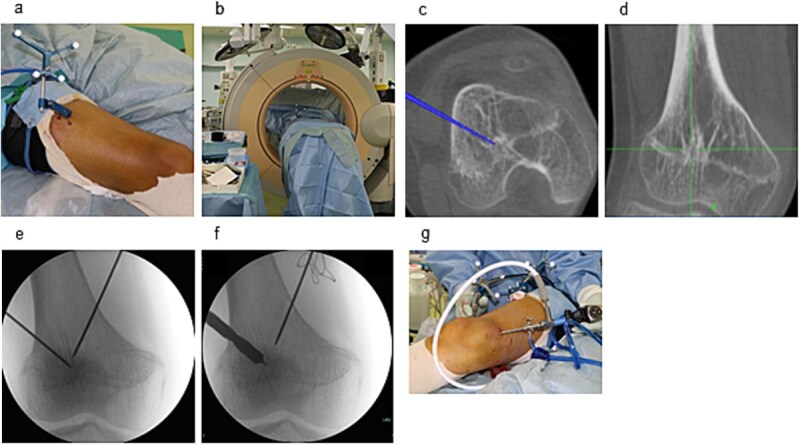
Intraoperative setup and portal creation. (a) Two 3-mm pins were inserted into the femoral diaphysis to secure the tracker of the Stealth Station™ system. (b) Intraoperative CT was performed using the O-arm™ system and integrated with the navigation system. (c) The location and trajectory to the physeal bar were identified using navigation. (d) Two 1.6-mm Kirschner wires were inserted from the medial and lateral aspects toward the physeal bar under fluoroscopic guidance. (e) Drill portals (8 mm) were created along the wire tracts. (f) A 4-mm arthroscope was inserted through one portal for endoscopic visualization.
